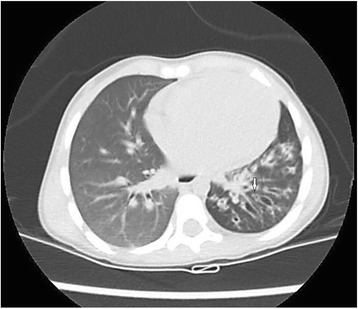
HRCT of a 3-year-old child showing several cylindrical bronchiectasis of the lower left lobe. The arrow indicates the classic “signet ring sign”
